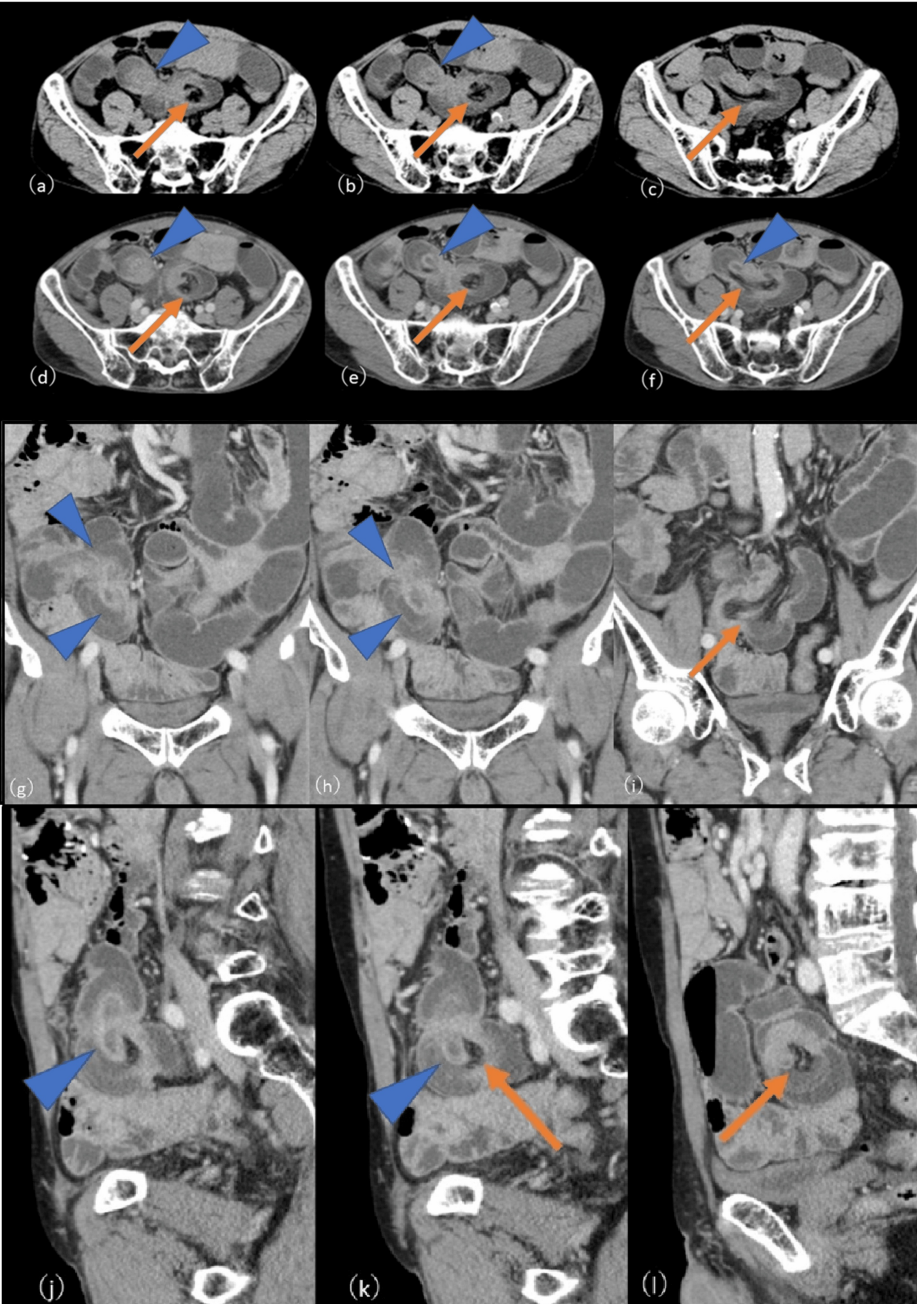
(a-c): Unenhanced CT (axial), (d-f): Contrast-enhanced CT (axial), (g-i): Contrast-enhanced CT (coronal), (j-l): Contrast-enhanced CT (sagittal) Abdominal CT revealing ileo-ileal intussusception in the distal ileum, with mesenteric fat trapped between the bowel loops (arrows). A high-attenuation, round mass is visualized at the leading edge of the intussusception, which is continuous with a tubular structure and exhibiting poor contrast enhancement (arrowheads).
